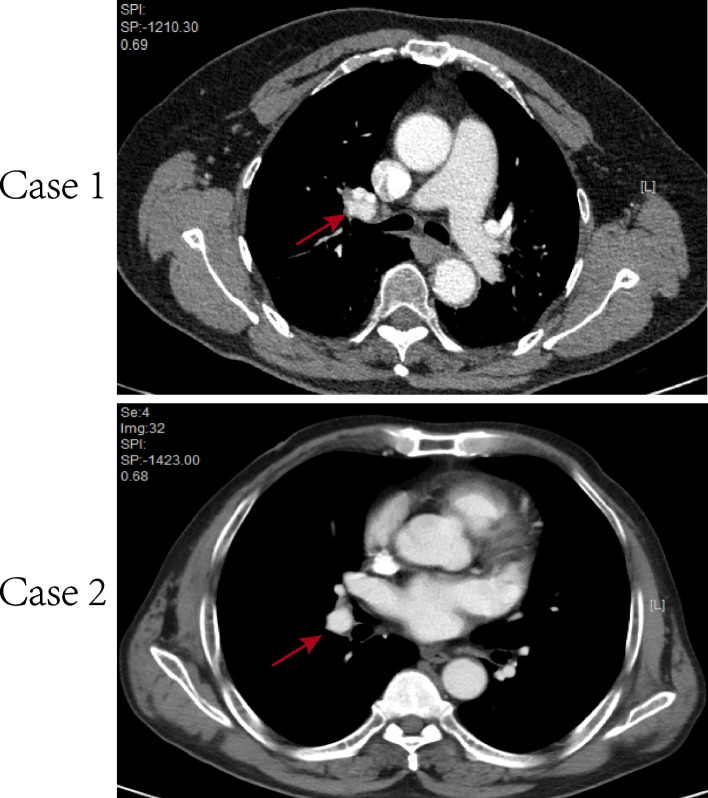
Imaging characteristics of two patients. A, B CT showed a well-defined nodule in the lower lobe of the right lung, measuring approximately 3.5 × 3.3 × 2.8 cm and 4.4 × 4.1 × 3.5 cm
